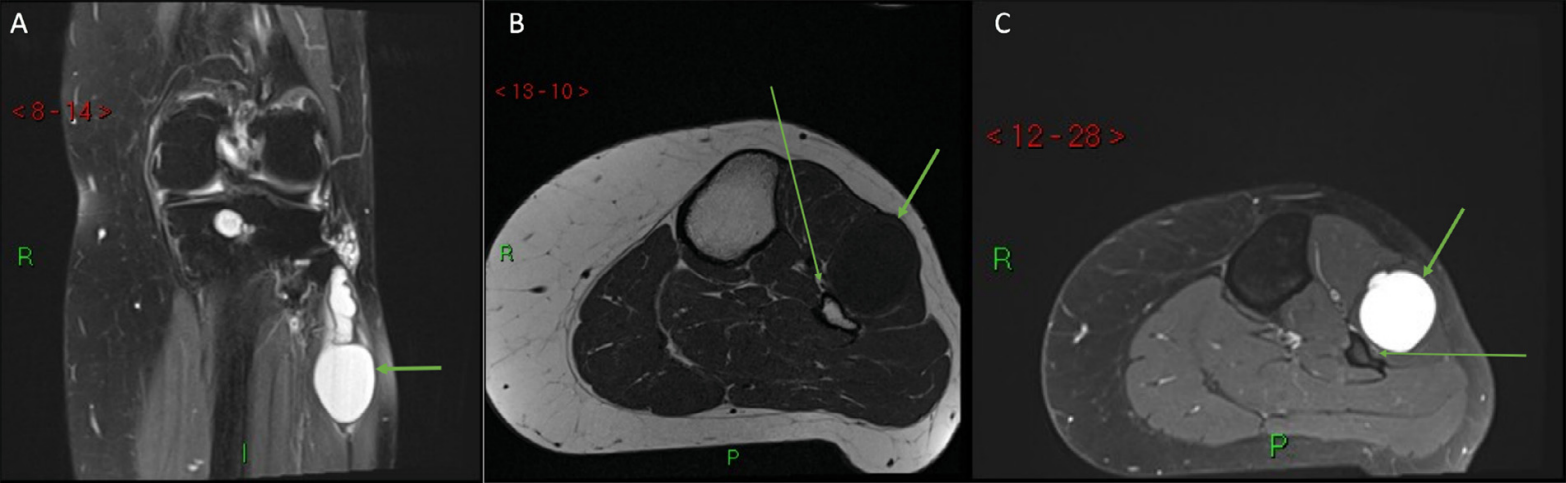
(A, B, C): Left knee MRI in coronal Proton Density Fat Suppressed (A), axial T1-weighted (B) and axial Proton Density Fat Suppressed (C) showing a multi-loculated ganglion cyst anterolateral to the proximal fibula (thick arrow) causing peroneal nerve compression (thin arrow). R is right; I is inferior; P is posterior.
